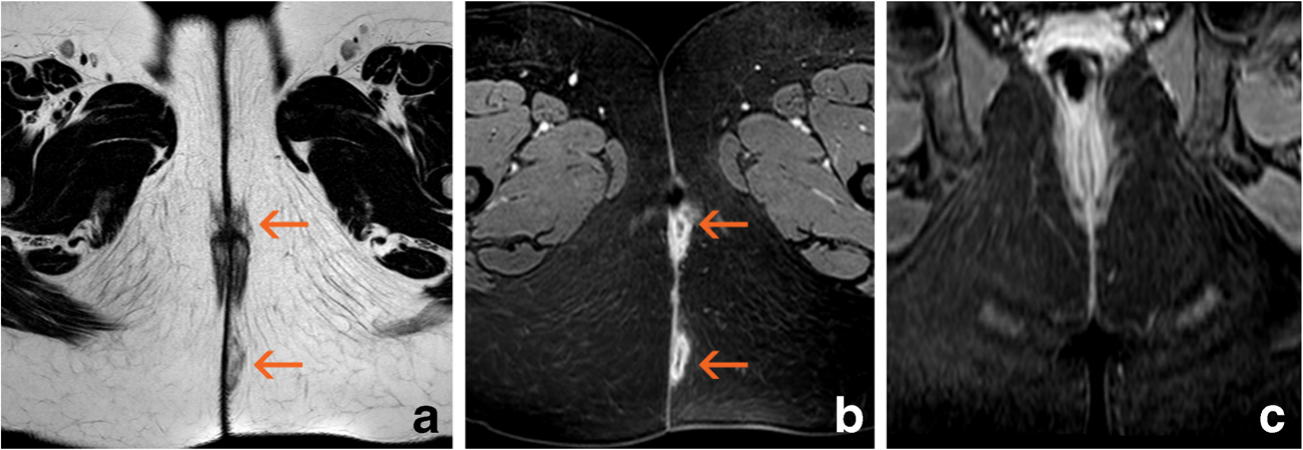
Hidradenitis suppurativa. Skin and subcutaneous abscesses (arrows) are present in the anal cleft without anal involvement. a Oblique-transverse T2-weighted image. b Post-gadolinium oblique-transverse fat-supressed gradient echo T1-weighted image. c Post-gadolinium oblique-coronal fat-supressed gradient echo T1-weighted image
